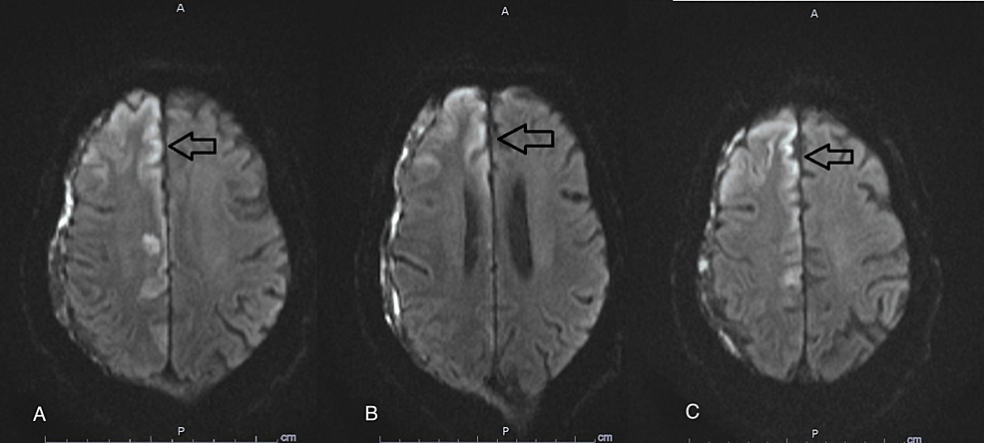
MRI diffusion trace sequence Magnetic resonance imaging (MRI) of the brain diffusion trace sequence showing evidence of diffusion restriction/cortical ribbon affecting the right frontal and paramedical regions (arrows in A, B, and C)
